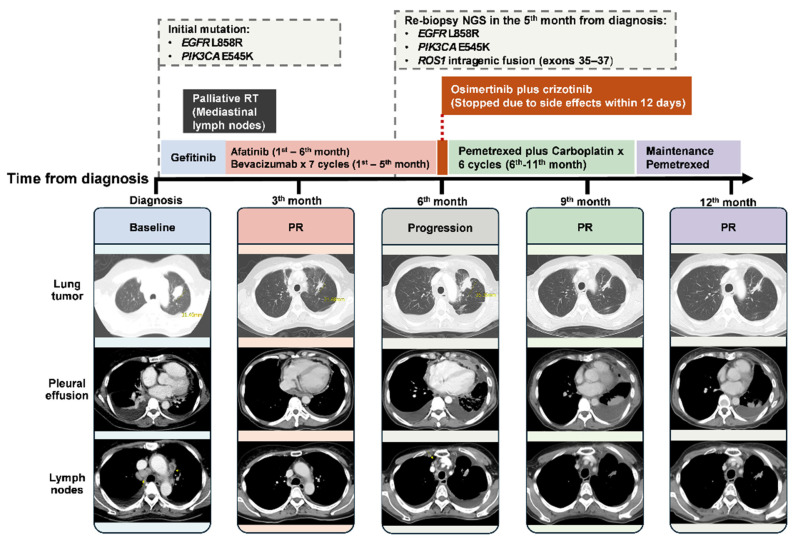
Timeline of Case 2 with successful platinum-based chemotherapy salvage following dual TKI intolerance in *EGFR*-mutant NSCLC with acquired *ROS1* rearrangement. Serial chest CT images show changes in the target lung lesion, pleural effusion, and lymph nodes. The lung tumor measured 31 mm at diagnosis, decreased to 22 mm at month 3, increased to >35 mm at progression at month 6, and decreased to 17 mm and 16 mm after platinum-pemetrexed chemotherapy at months 9 and 12, respectively. Target lesion measurements and response assessments are shown according to RECIST v1.1 where evaluable. PR: partial response; NGS: next-generation sequencing.
